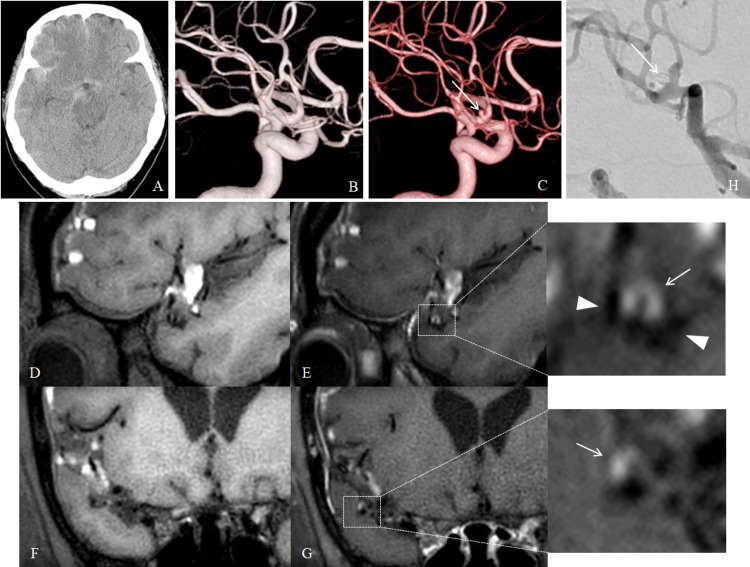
Case 2 (A) Computed tomography shows subarachnoid hemorrhage in the right Sylvian fissure. (B) Initial digital subtraction angiography did not detect any vascular lesions. (C) Second digital subtraction angiography shows a small aneurysm (arrow). (D, E) Sagittal and (F, G) coronal views of magnetic resonance vessel wall imagings (D, F) pre-contrast and (E, G) post-contrast administration show enhancement of the aneurysm wall (arrow: enhancement, arrow heads: middle cerebral arteries). (H) Digital subtraction angiography after endovascular coil embolization shows disappearance of the aneurysm (arrow).
